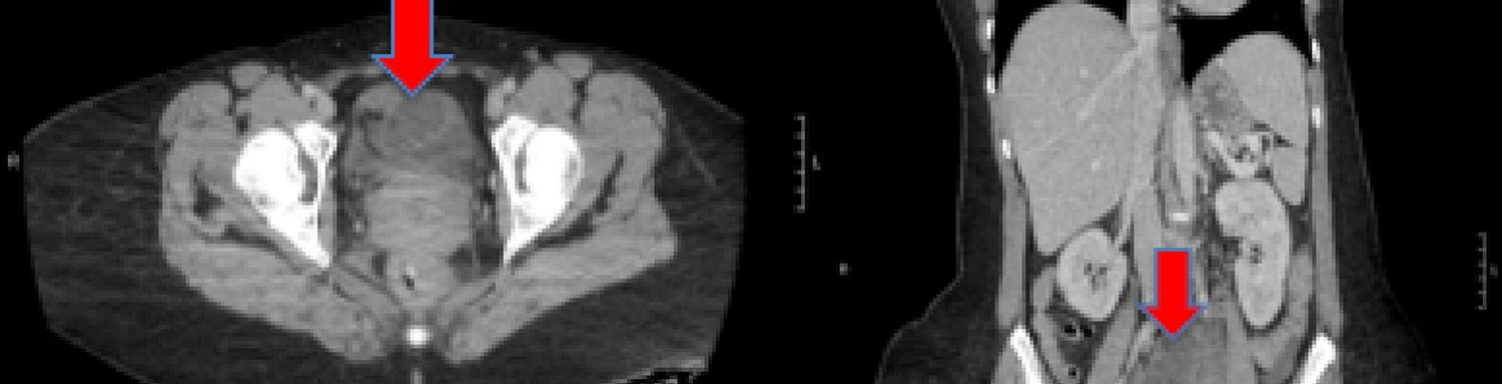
CT scan of the abdomen with IV contrast.* It showed an annular lesion in the Jejunum (Image on the left with the red arrow at transition point), signaling as the cause of SBO (proximal dilation of SB loops as pointed by the arrow at the right image)
